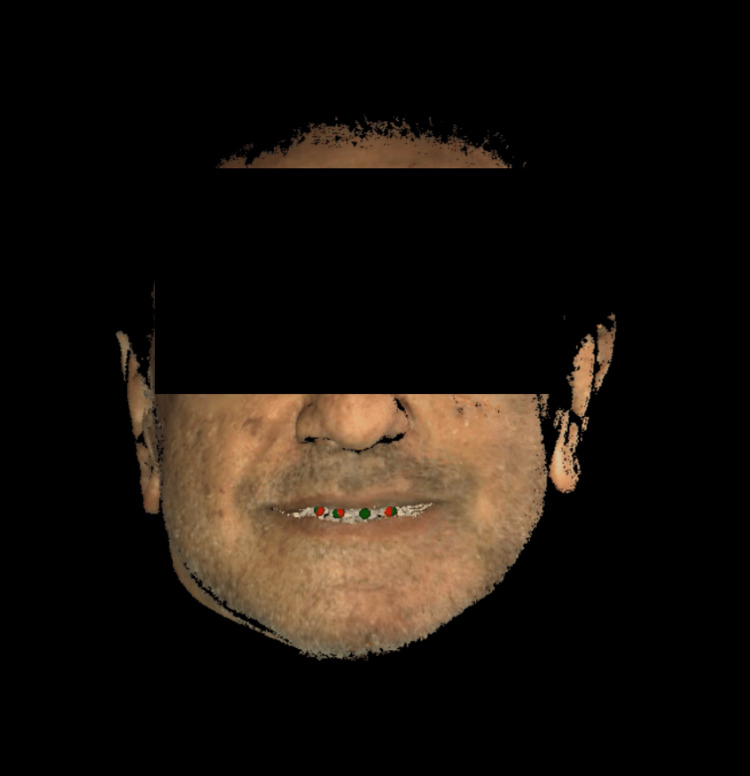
Integration of face scanning data. The maxillary anterior teeth are used as reference landmarks for the alignment with the CBCT and IOS data CBCT: Cone Beam Computed Tomography, IOS: Intraoral Scanning
